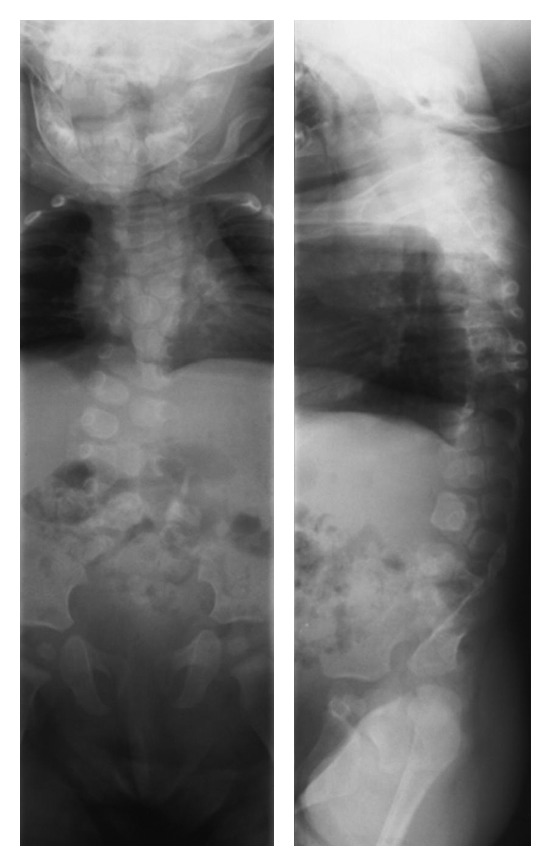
Anteroposterior (AP) and lateral radiographs obtained at 6 months of age demonstrating multiple, asymmetric vertebral segmentation defects of the thoracolumbar vertebra accompanied by asymmetric rib deformities, partial distal sacral agenesis, and fused cervical vertebrae.
